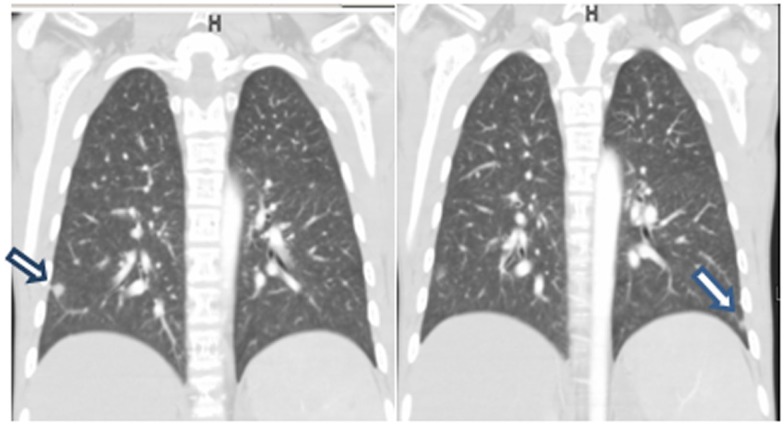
Coronal CT images showing AVM on right and left side (arrow).
